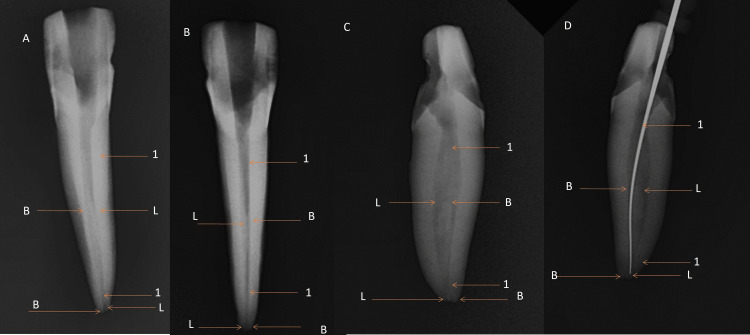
Multiangle radiographs for Vertuccii type VII (1-2-1-2) anatomy in 32 A) labial side; B) lingual side; C) mesial side; D) distal side L: lingual canal; B: buccal canal; *1: merging of two canals.
